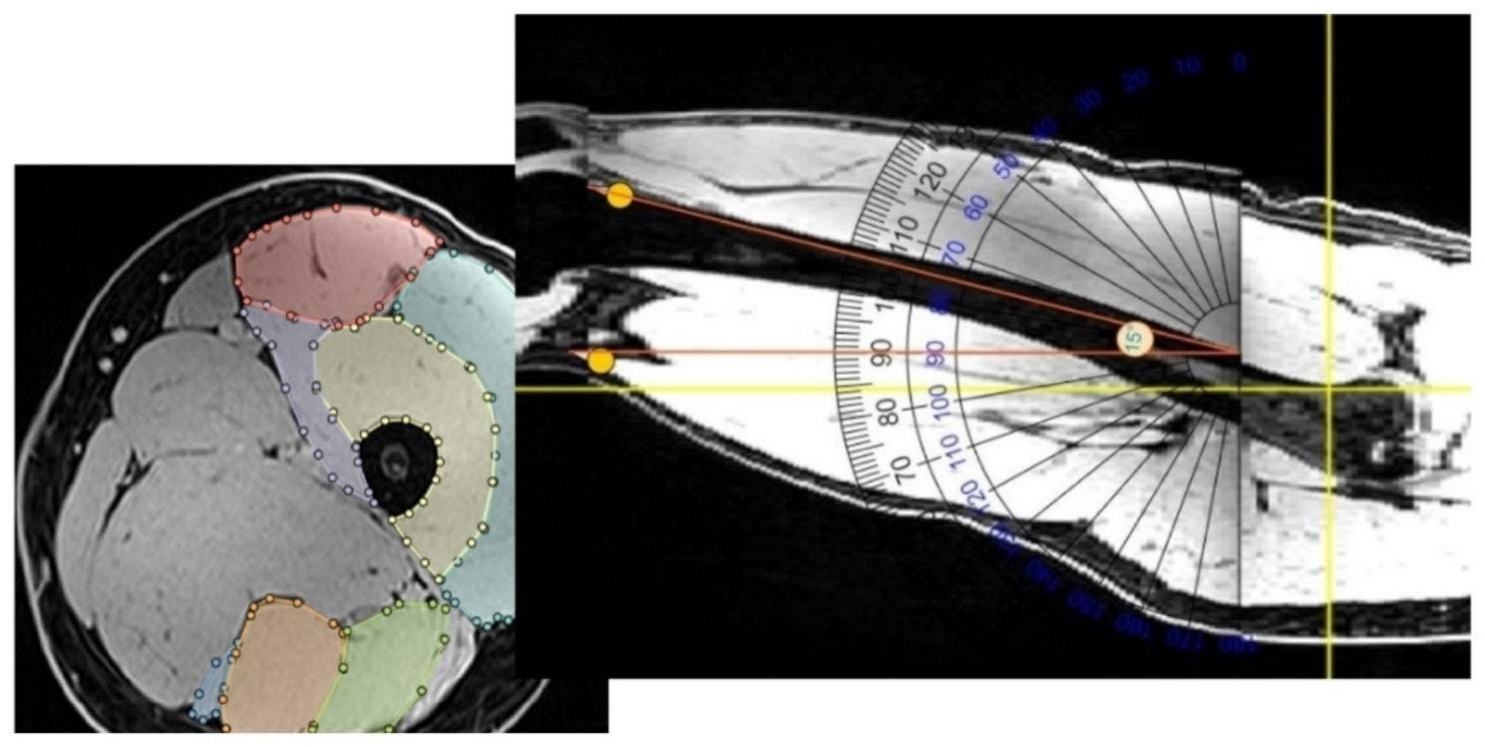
MRI-based segmentation of the cross-sectional shape of the quadriceps and the hamstring muscles in each image slice (left). Digital goniometer to assess the angle between the anatomical axis of femur and the transversal imaging plane (right)
